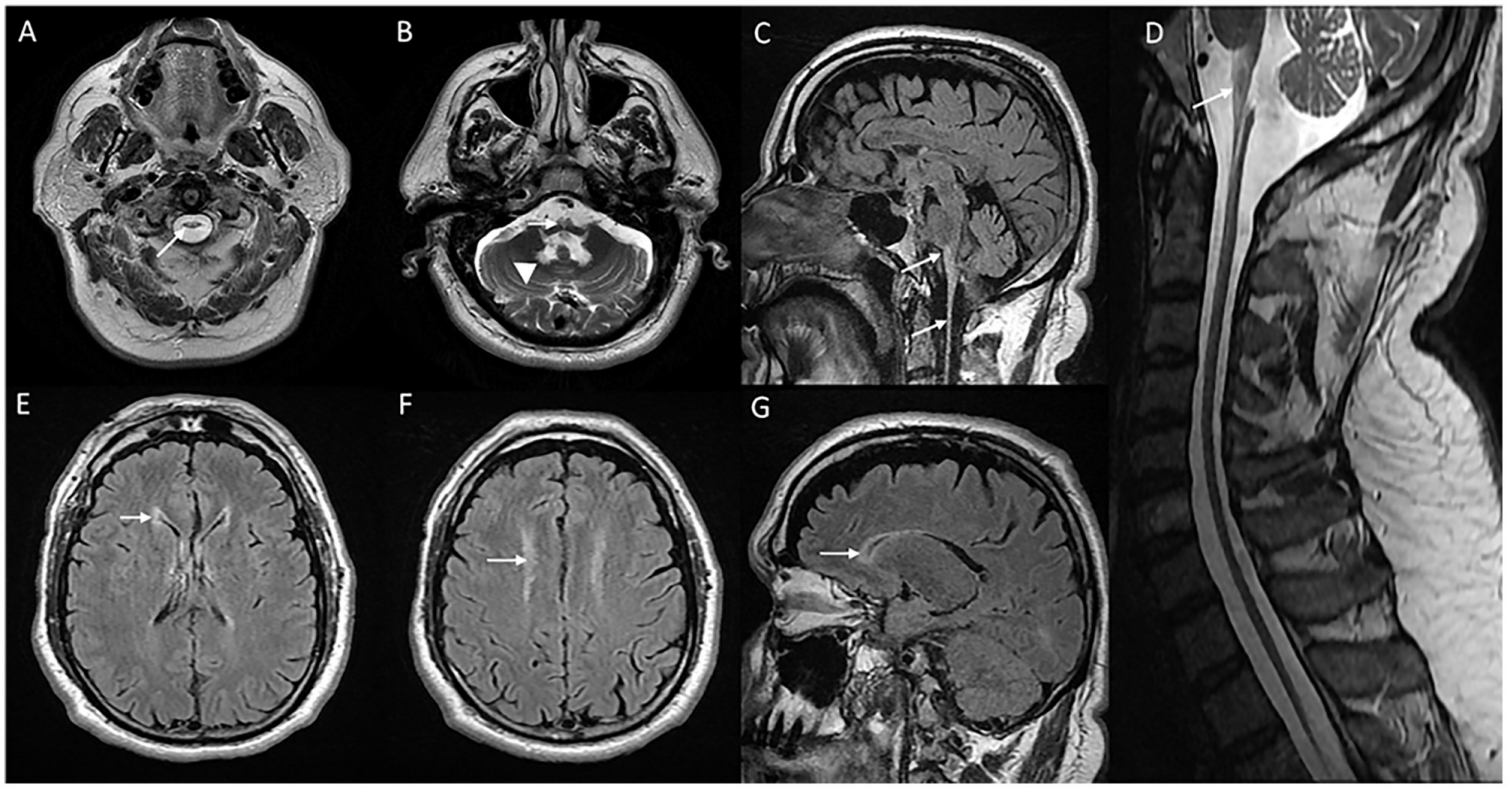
MRI of brain and cervical cord in adult-onset Alexander disease. T2W axial sections with marked atrophy and intrinsic signal changes in the upper cervical cord (A) and lower medulla (B) along with symmetric signal changes in the dentate hilum of the cerebellum [arrowhead in (B)]. T2W sagittal FLAIR imaging demonstrates signal changes and atrophy of the medulla and upper cervical cord with a “tad pole” appearance (C). T2W sagittal spine imaging (D) reveals diffuse atrophy of the cervical cord. T2W FLAIR axial (E, F) and sagittal (G) demonstrate signal changes along the wall of lateral ventricles predominantly along the frontal horns.
